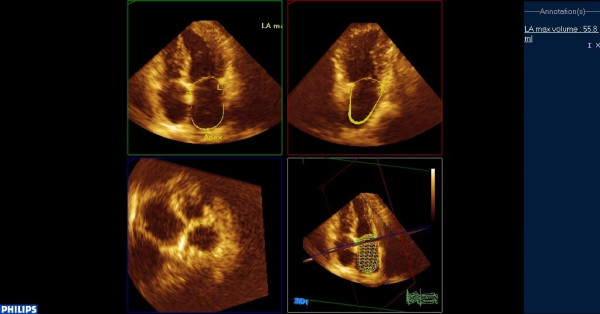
Assessment of LA volumes using RT3DE. Automatic border detection is obtained marking 5 reference points in the apical 2- and 4-chamber views (upper panel) and the LA 3-dimensional model is provided by the software (lower panel). The figure captures an end-systolic frame, from which left atrial maximum volume is derived.
